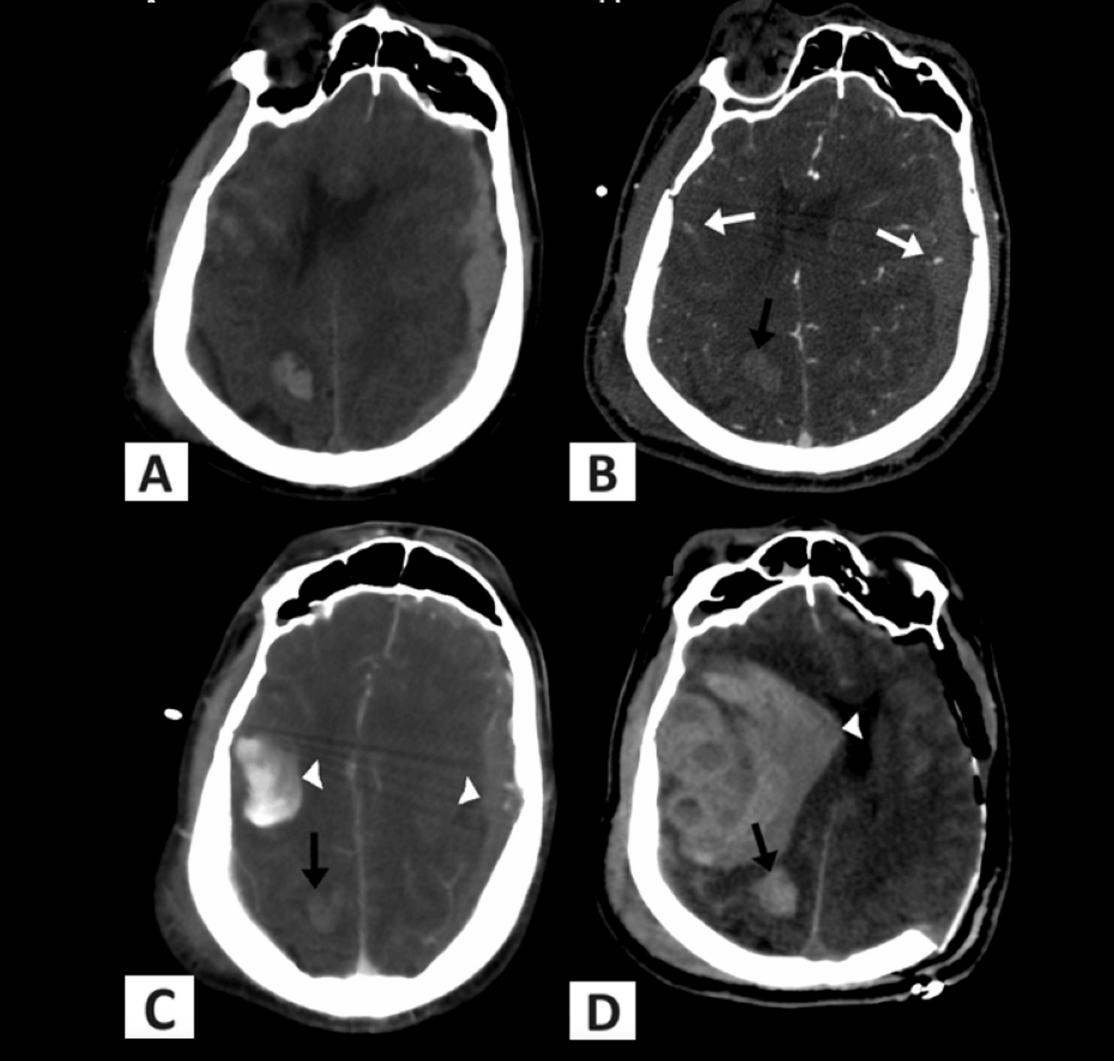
Pre- and postop neuroimaging (A) The initial NCCT of the head shows a large left convexity mixed density acute SDH, resulting in a mass effect on the underlying brain, with a rightward midline shift, and subfalcine herniation. Extensive SAH and multifocal cortical contusions involving the right parietal, bilateral frontal, and temporal lobes are noted. (B) The baseline CTA shows a left SDH and a right temporal contusion with two small foci of contrast extravasation consistent with spot signs (white arrows). It also demonstrates a right parietal contusion without a spot sign (black arrow). (C) The 90-second delay CTA shows marked expansion of both the right temporal contusion and of the left convexity SDH (arrowheads), but with no right parietal contusion expansion (black arrow). (D) Immediate postoperative NCCT demonstrates the increased size of the left frontal contusion (arrowhead) as predicted by the spot sign. There is no SDH in the left convexity due to the patient’s history of craniotomy. No parietal contusion expansion is noted (black arrow). NCCT: Non-contrast computed tomography; SDH: subdural hematoma; CTA: Computed tomography angiography.
